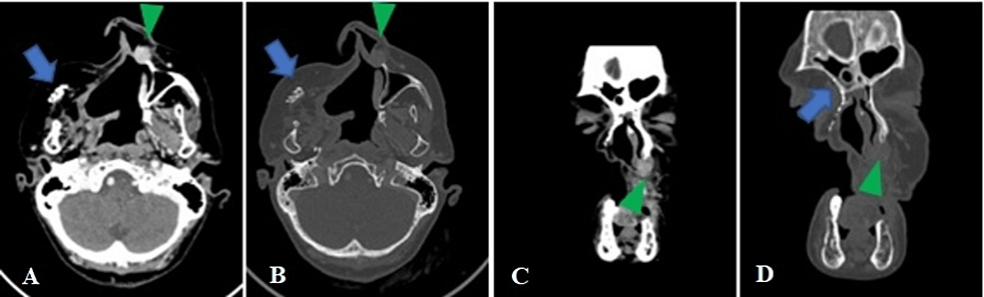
Seven years after the initial treatment. Contrast-enhanced CT after extended maxillectomy (A-D), with reconstruction (arrow). Circumscribed nodular lesion (arrowhead) located in the left nasal septum.
